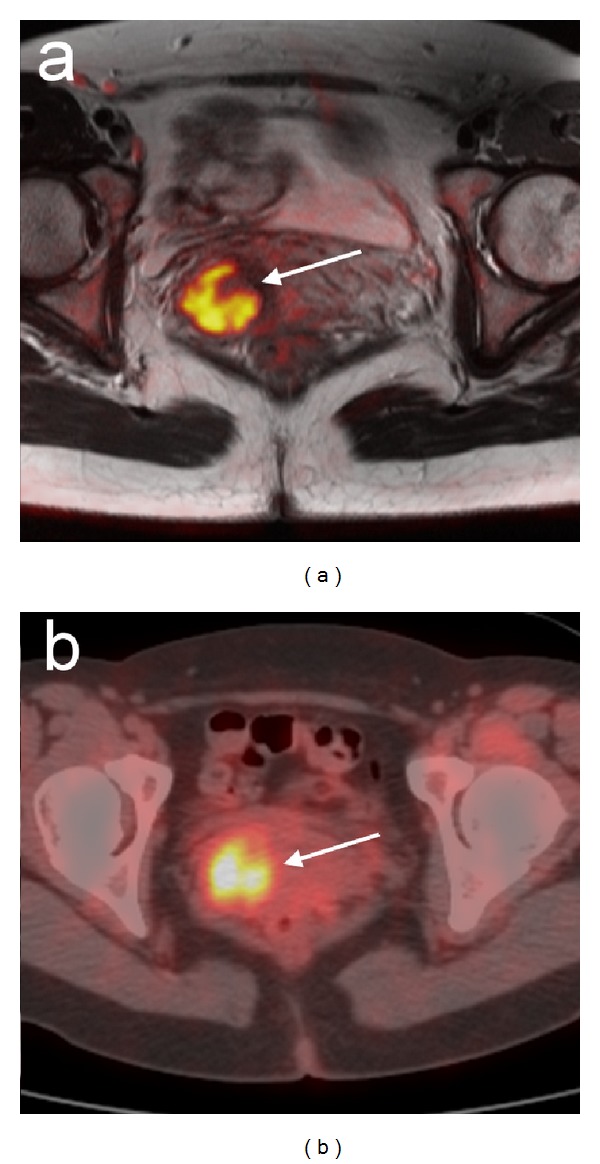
An example of PET/CT and MRI in the female pelvis. A 43-year-old female patient with a primary well-differentiated adenocarcinoma of the uterine cervix. Primary cervical tumor is highlighted (arrow) and well correlated in (a) diffusion-weighted MRI and (b) 18F-FDG PET/CT. Reprinted with permission from [56]. Copyright 2008 Springer-Verlag.
